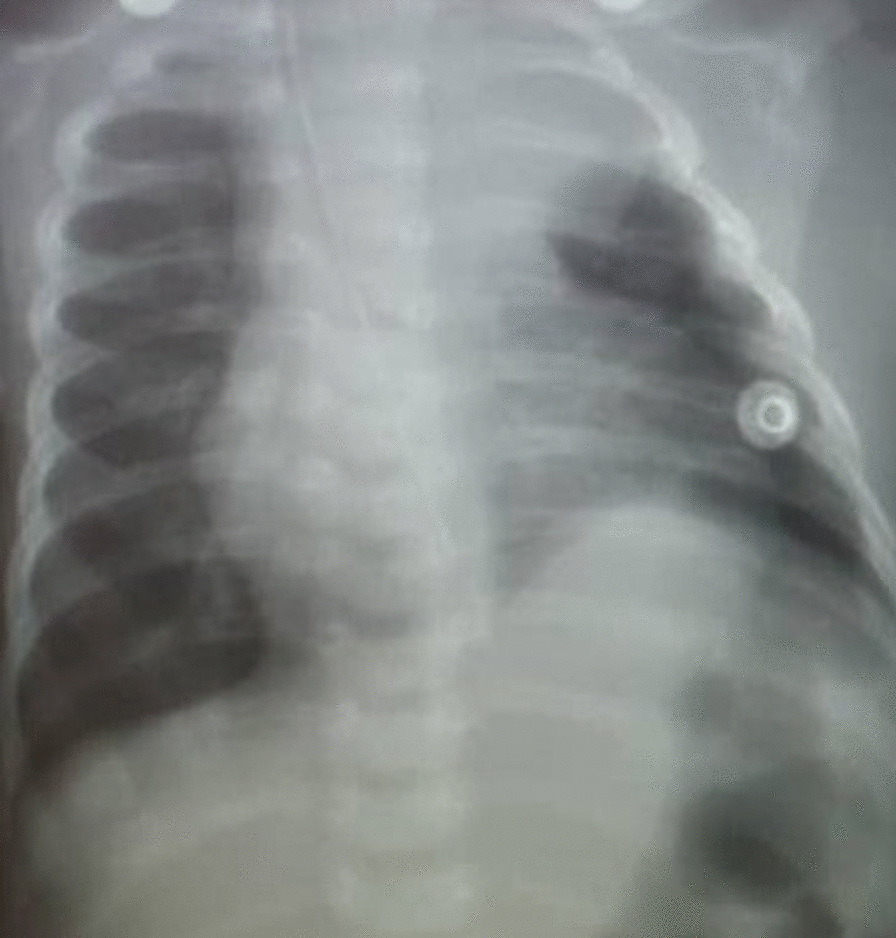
Chest X-ray done 48 h post-surgery, showing left upper lobe lung hypoplasia
